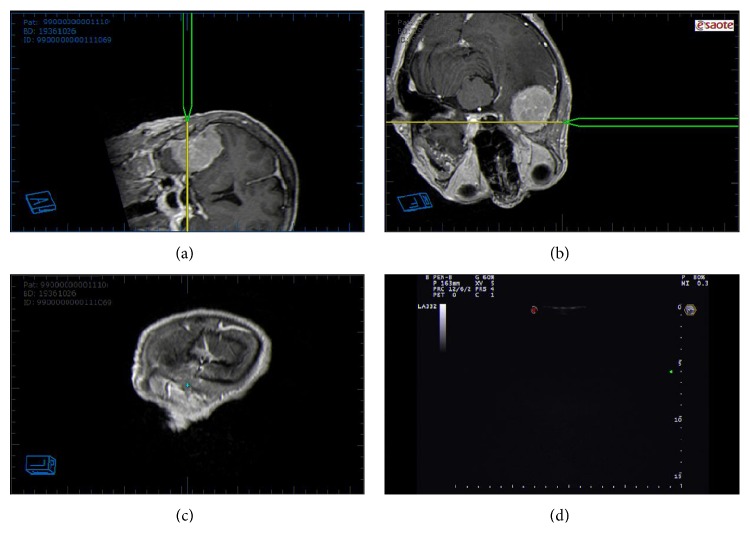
Craniotomy planning. In this screenshot, the pointer position is represented in three reconstructions of preoperative MRI. Using this feature, it is possible to plan the craniotomy site. In the lower-right box is visualized real-time ioUS that does not give any information because of bone shielding.
